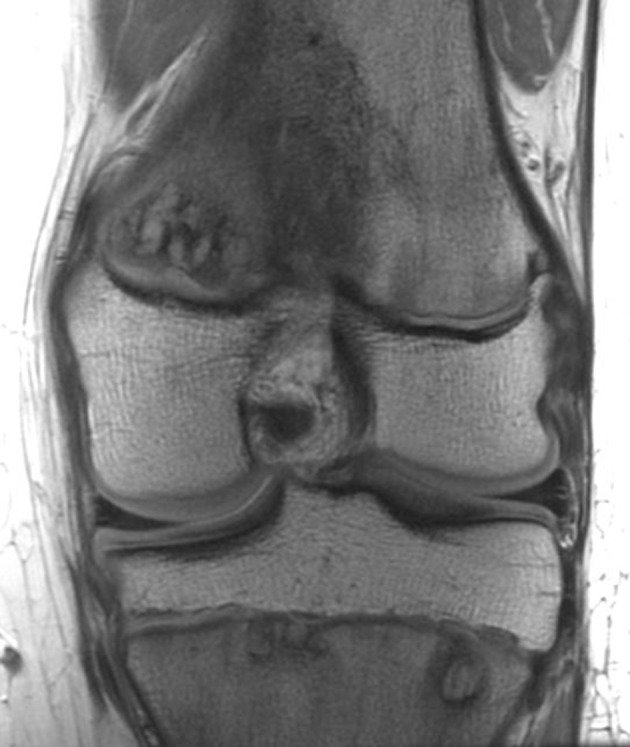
Multiple metaphyses affected in the left knee. Coronal proton density (MRI) image shows multiple areas of abnormality extending from the physes into the metaphyses of the distal femur and proximal tibia.
